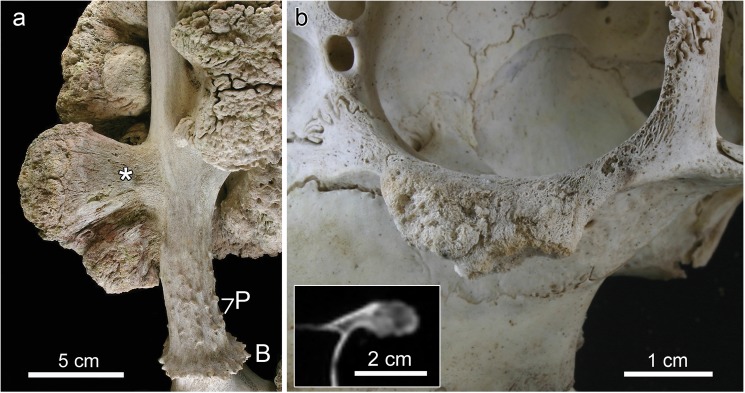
Close-up views of exostoses arising from the right antler (a) and the left zygomatic bone (b) of the white-tailed buck (*Odocoileus virginianus*). (a) Rostral view of the right antler showing the smaller bilobular exostosis and portions of the more distally located larger one. Note the smooth surface of the stalk (asterisk) and the rugose/nodular surface of the peripheral portion of the mass. B: burr at antler base; P: small protuberances (‘pearls’) of the antler surface. (b) Lateral view of the left zygomatic bone with broad-based exostosis. The insert shows a CT image demonstrating continuity of spongiosa and cortex of the exostosis with those of the parent bone.
